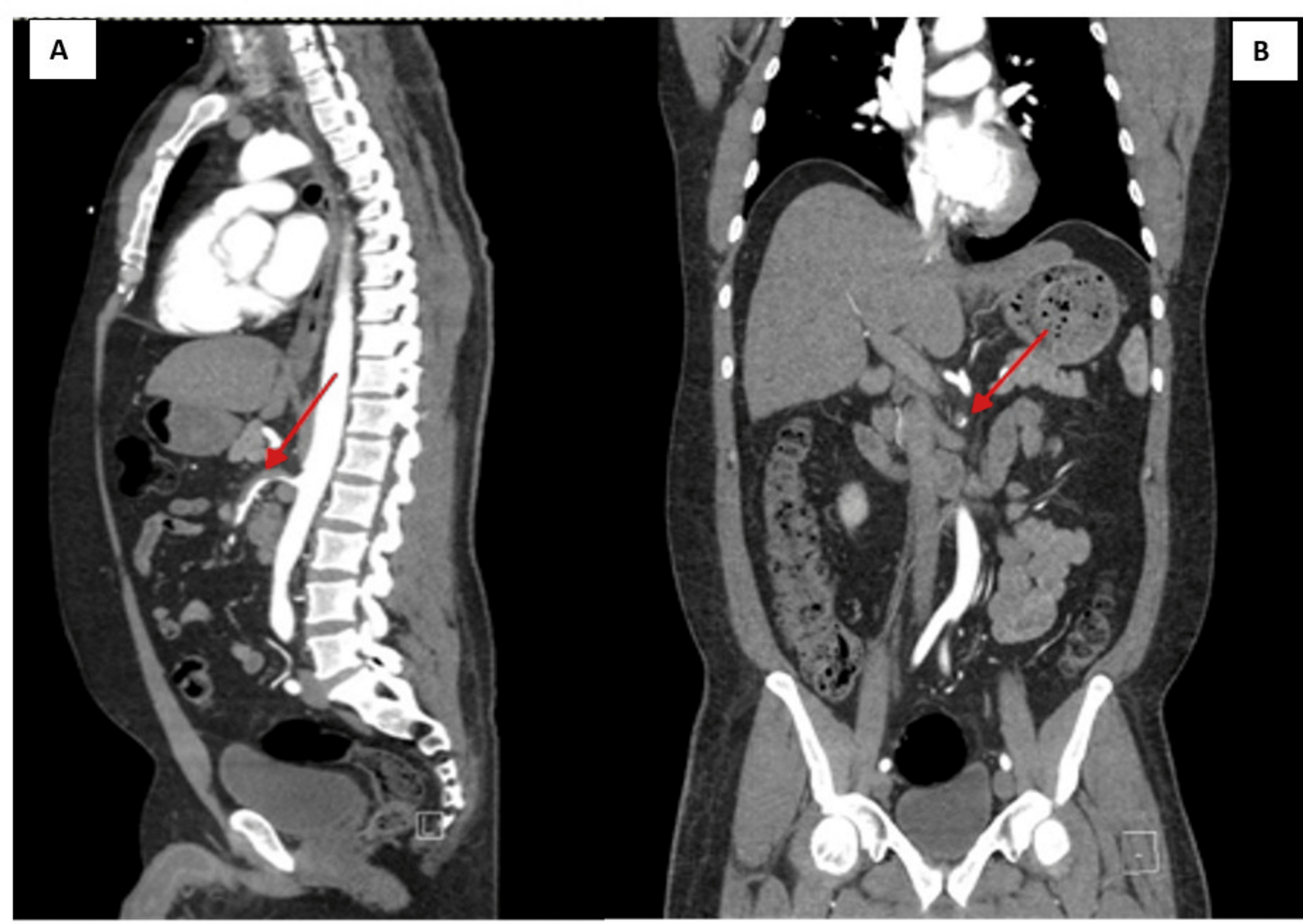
Sagittal (A) and coronal view (B) of CT angiogram of the abdomen and pelvis with superior mesenteric artery (SMA) dissection just after the aortic takeoff, demonstrating thrombus (red arrow) in the false lumen of the SMA dissection.
